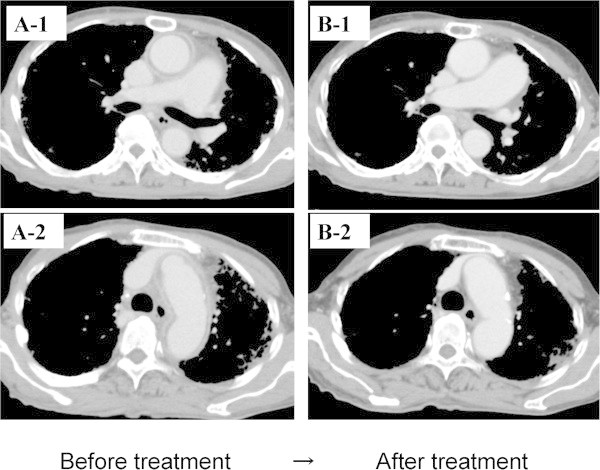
Thoracic contrast-enhanced CT findings of aortitis before and after steroid therapy. A-1 and A-2. Before steroid therapy, the thoracic contrast-enhanced CT demonstrated thickening of the aortic wall at the level of the ascending aorta and aortic arch, brachiocephalic artery, and left subclavian artery with a contrast effect. B-1 and B-2. After steroid therapy, the thickening of the aortic wall had disappeared completely.
